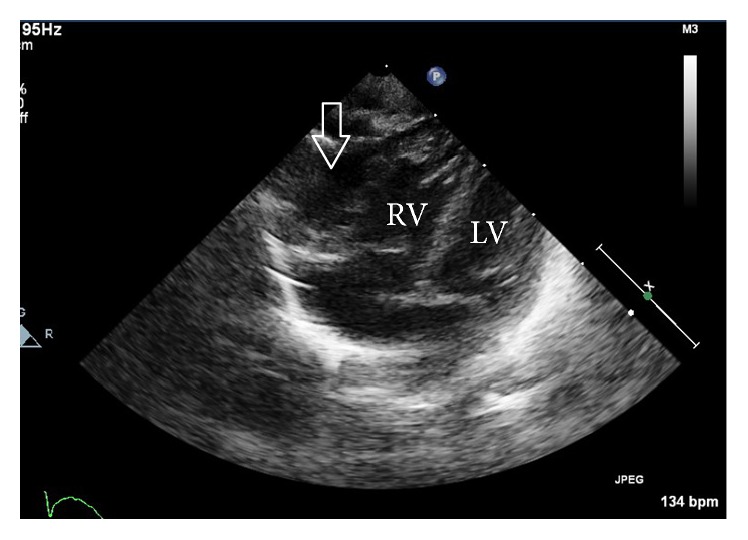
Postnatal ultrasound: four-chamber view showing the diverticulum arising from the right ventricle. LV, left ventricle; RV, right ventricle. Arrow indicates the diverticulum.
